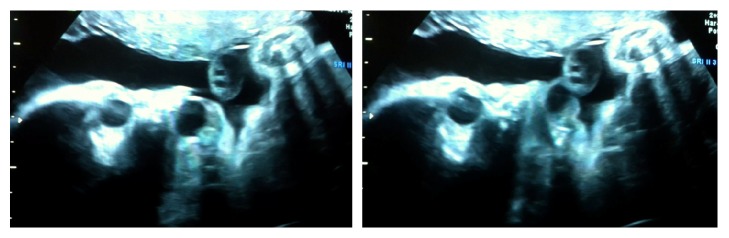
Ultrasound image showing how the tumor moves with the tongue movements and it is completely out of the fetal mouth.
